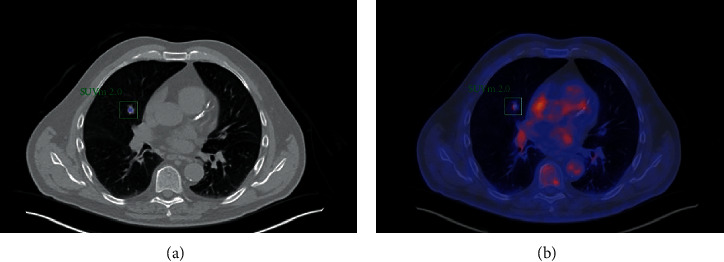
(a) Computed tomography image with the corresponding (b) positron emission tomography image showing 16 mm right middle lobe lung lesion (FDG avid, SUV max 2.0).
